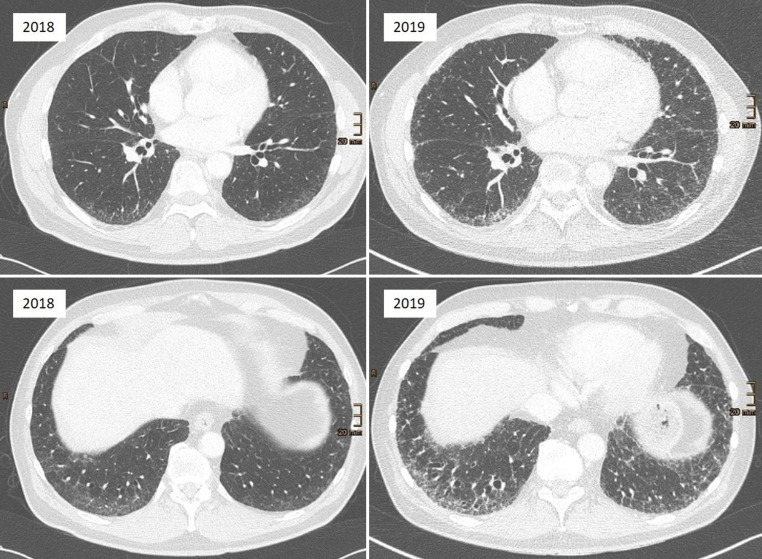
Beispiel für ein Voranschreiten von interstitiellen Lungenanomalien (ILA) zur interstitiellen Lungenerkrankung (ILD) binnen eines Jahres. 2018 zeigen sich in einem Kontrastmittel-unterstützten CT als Zufallsbefund basal betonte subpleurale Retikulationen und Milchglas sowie geringe posterobasale Traktionsbronchiektasien („subpleural fibrotic ILA“ nach Fleischner Society). 2019 zeigt sich die Progression zu einer ILD mit zunehmenden Retikulationen, Milchglas, Traktionsbronchiektasien und Lungenvolumenverminderung
